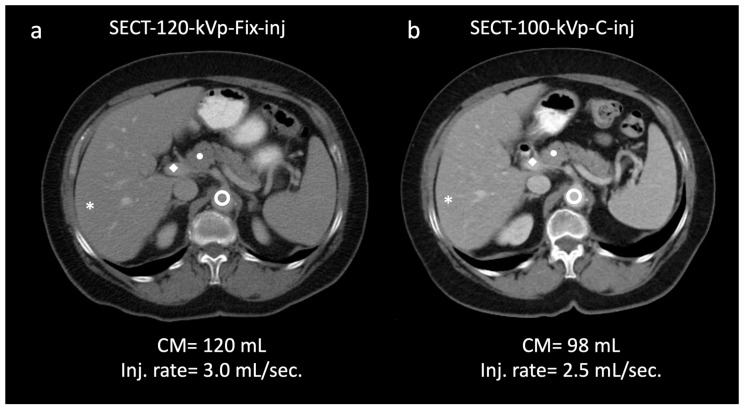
Clinical example of a 62-year-old male (93 kg). A 120 kVp single-energy CT (SECT) with fixed injection (120 mL) demonstrates the following attenuation (HU)/contrast-to-noise ratio (CNR) values: * liver; 97.6/19.6; ● pancreas; 83.8/16.7;
